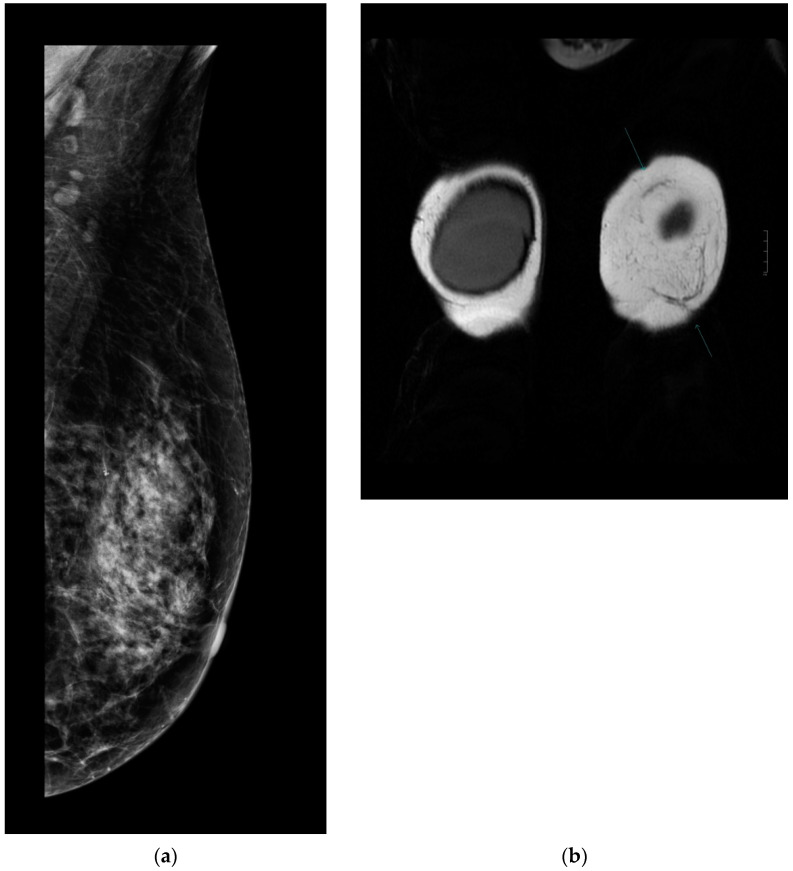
(a). Follow-up mammography after ULTRAPRO sling mastopexy. The mesh is not visible on the mammography images; therefore, it does not influence the oncological surveillance. (b). Arrows show the mesh on contrast-enhanced MRI. The mesh appears clearly/well depicted on the MR (coronal T2 weighted) images without disturbing the evaluation of the parenchyma.
